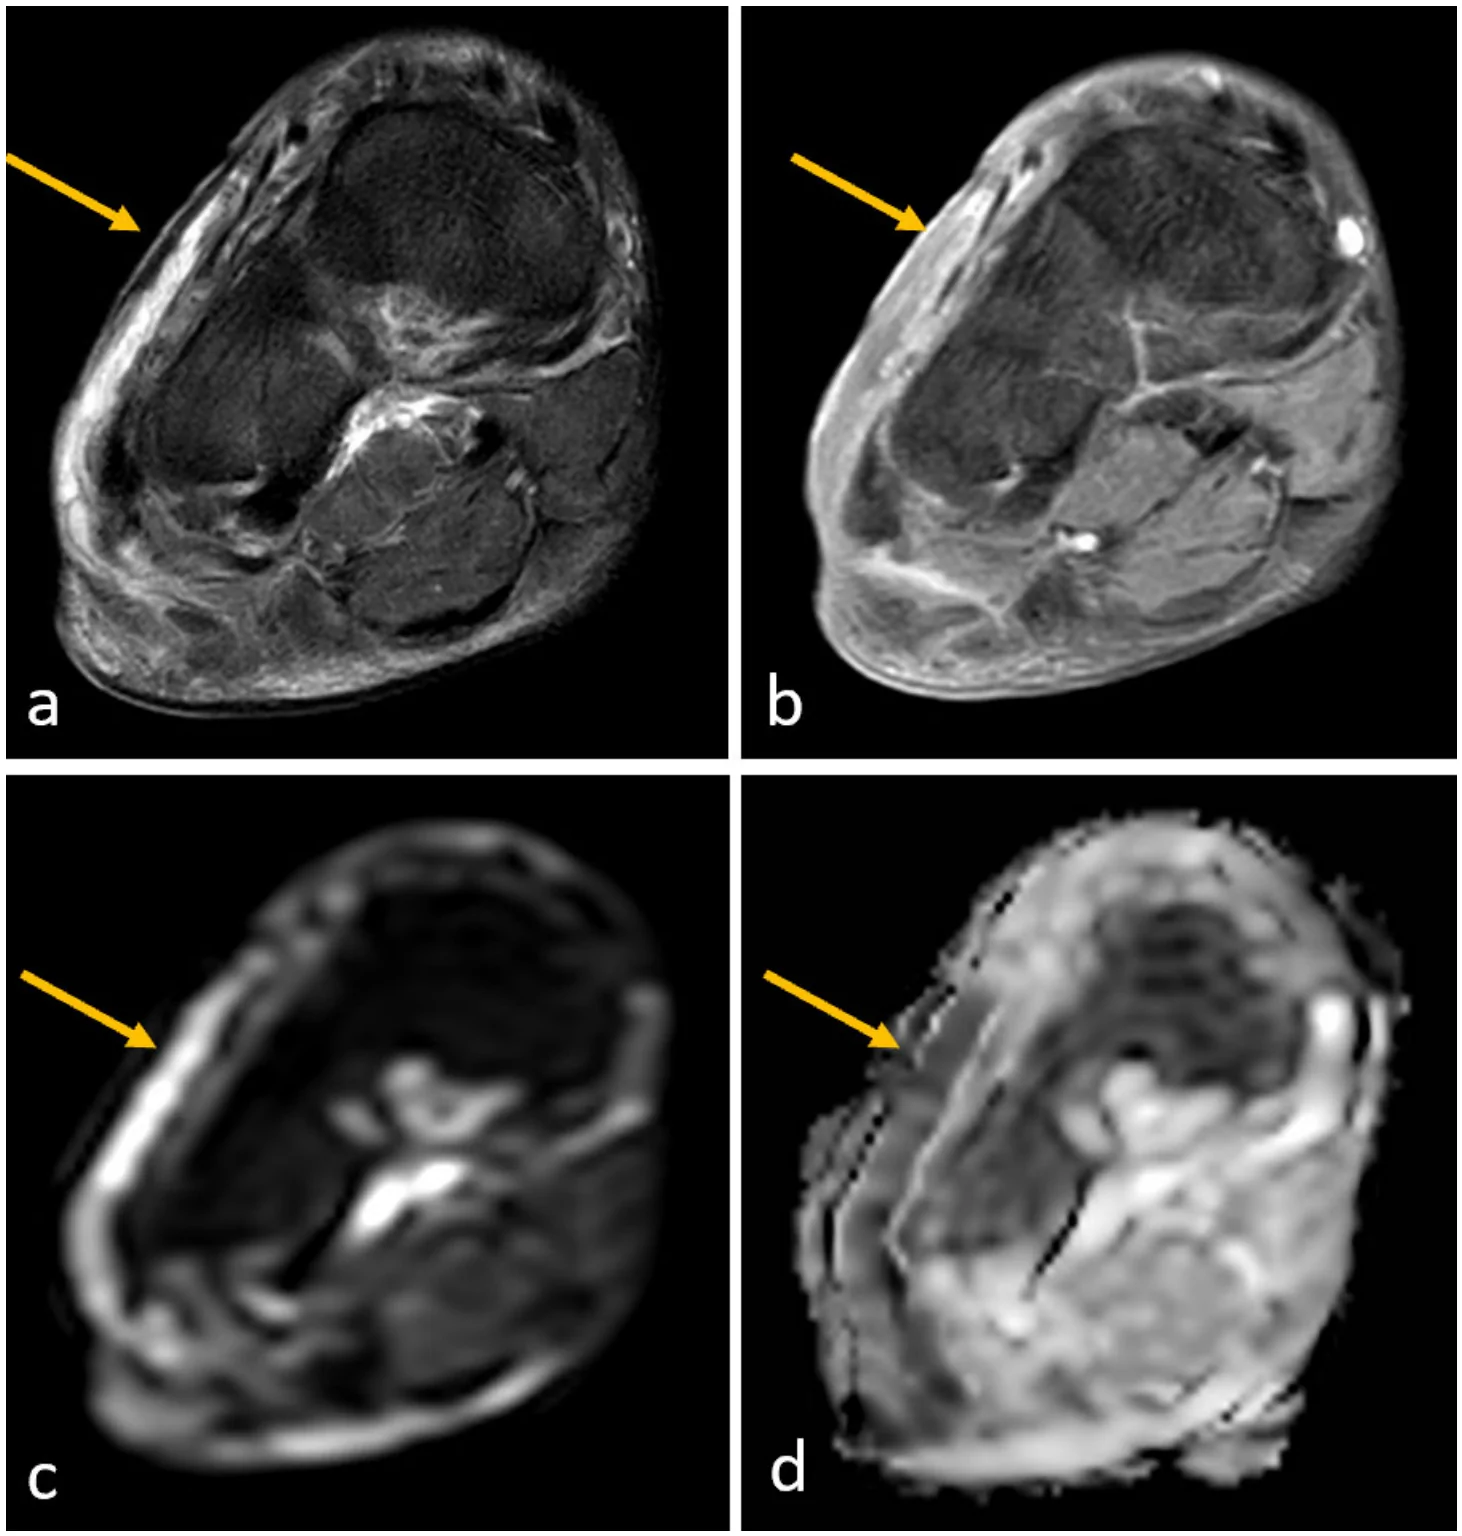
MRI showing improved assessment of an abscess on DWI (microbiology-proven). An ill-defined fat-suppressed T2-weighted hyperintense collection (a) is seen on the dorsal aspect of the foot and is not well appreciated on the T1-weighted post-contrast image (b). On DWI (c), the extent of the collection is better appreciated, with corresponding restricted diffusion on the ADC map (d) (orange arrows), suggesting that DWI provided better delineation of the collection than post-contrast imaging in this case. A single example cannot establish general superiority over contrast-enhanced imaging, and no comparative study data are cited here.
